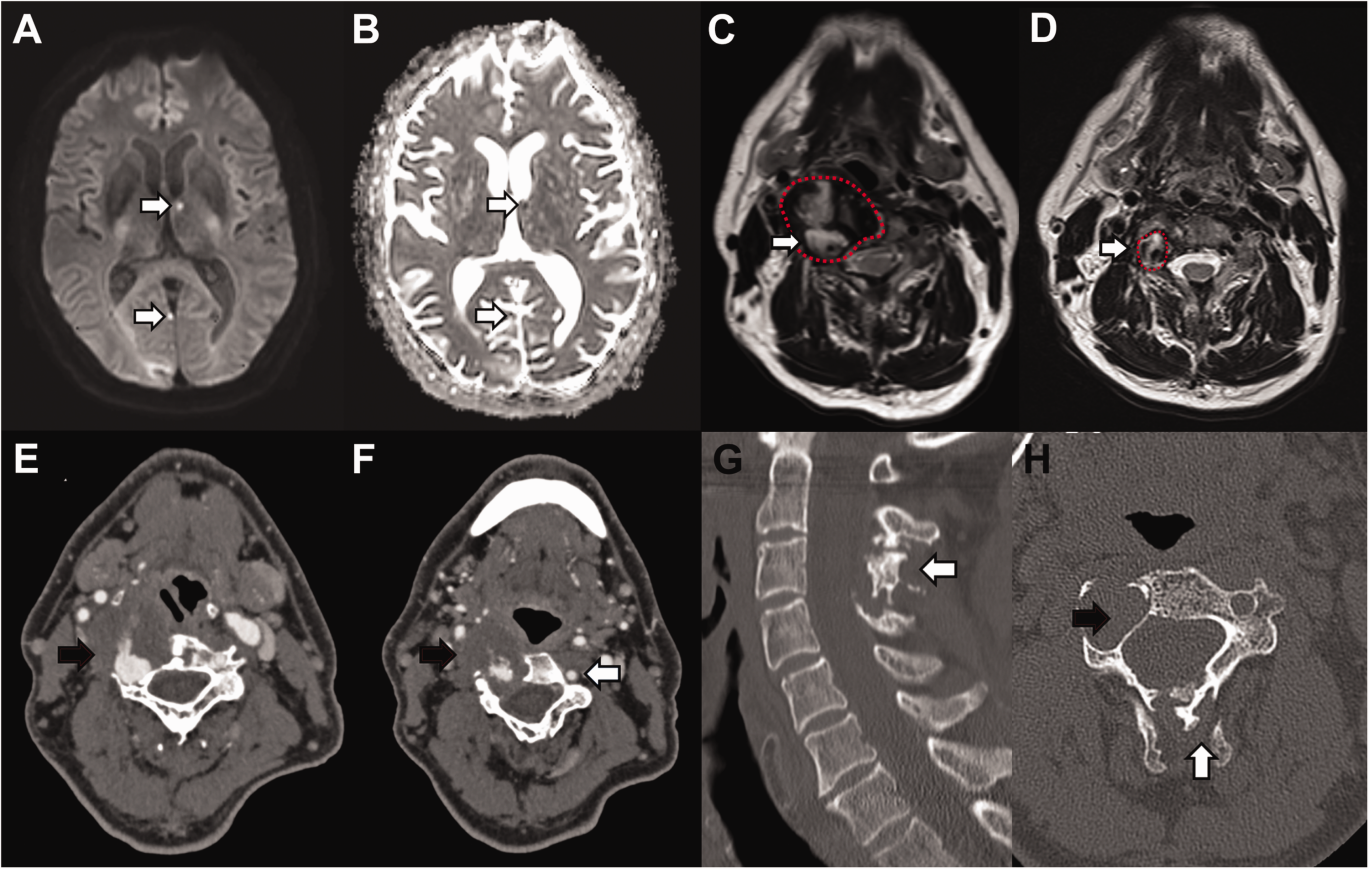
A and B: Multiple foci of restricted diffusion (white arrow), with corresponding low ADC value in the posterior circulation involving the right occipital lobe, left thalamus and cerebellum (not shown), in keeping with acute embolic infarction. Older non-restricting foci of embolic infarction were also evident on T2 sequences (not shown). C: T2 weighted sequence of the neck at the C4 vertebral level demonstrating an abnormal right vertebral artery (red broken line) with a central flow void and abnormal circumferential thick mixed T2 signal material (white arrow). Maximal diameter of the fusiform aneurysm was up to 35mm. D: T2 weighted sequence of the neck at the C4 vertebral level five years prior to presentation confirming significant growth (white arrow) of the right vertebral artery (red broken line). E and F: CT angiography demonstrated a grossly abnormal right vertebral artery measuring up to 5cm in diameter. The aneurysm demonstrated thick thrombus lining the lumen (black arrow) with areas of fissuring and crenulation. The contralateral left vertebral artery was of normal calibre and appearances (white arrow). G: Sagittal bone window reconstruction of CT demonstrates abnormal appearance to the spinous processes (white arrow) of C2 to C4 consistent with historic trauma. H: Axial CT at C3 level shows a grossly expanded right foramen transversarium consistent with a chronic process of fusiform aneurysm formation (black arrow).
